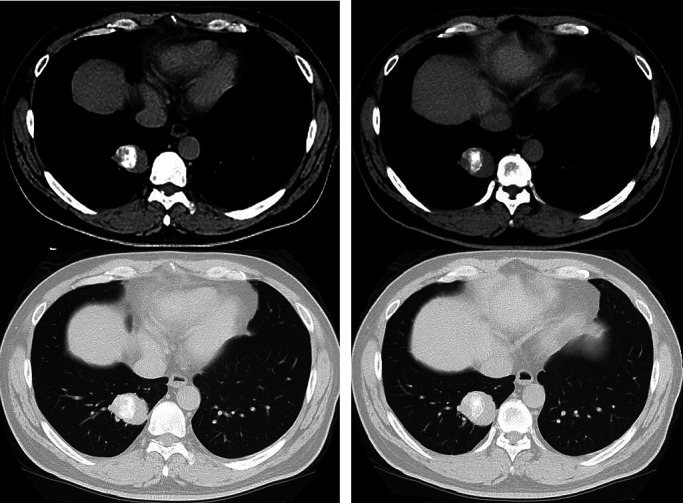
Chest CT showed a mass lesion, 4 cm in diameter, in the right lower lobe S10 with coarse
